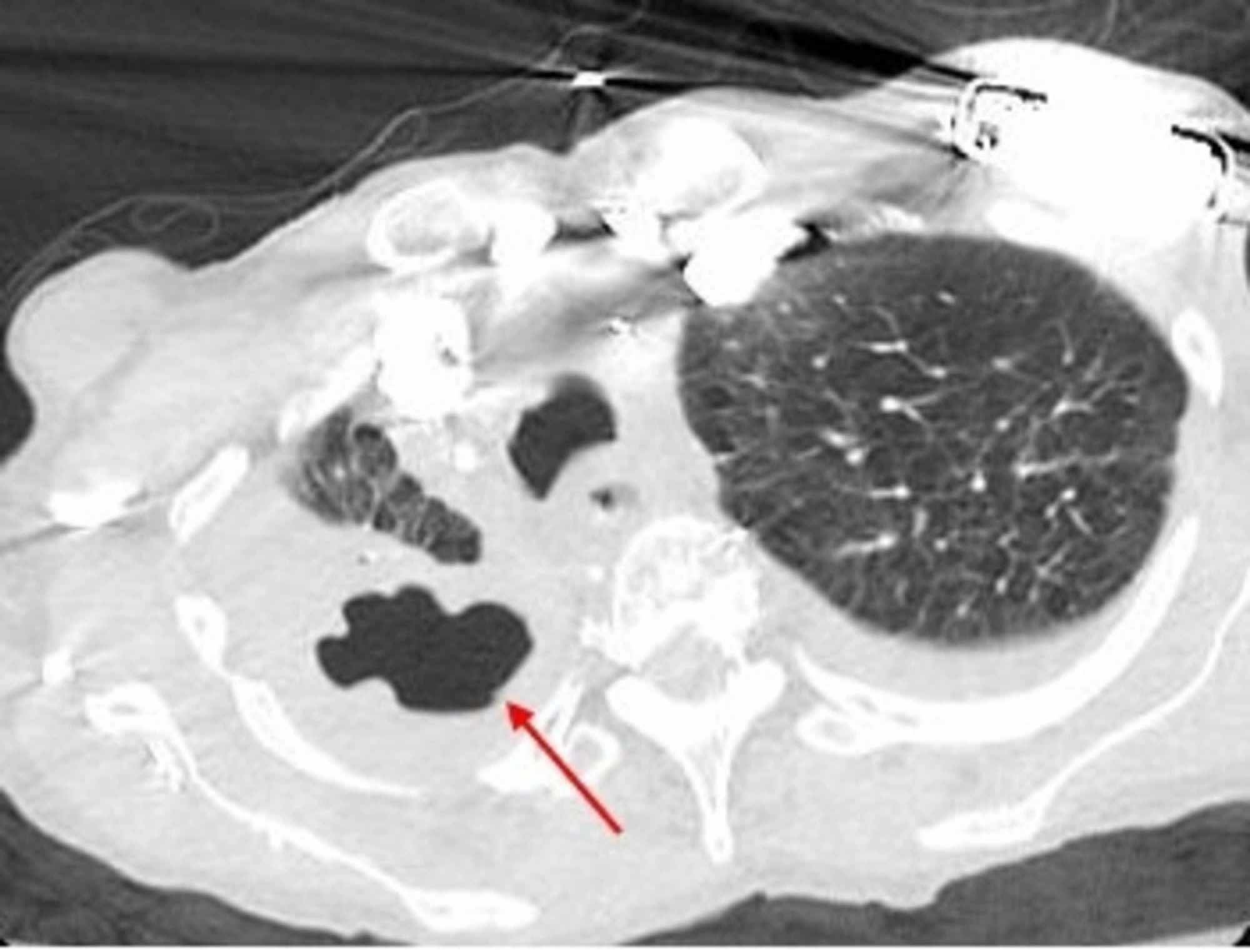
CT-thorax obtained at admission showing a gas-filled cavitary lesion (red arrow) surrounded by consolidation, in the upper lobe of the right lung
